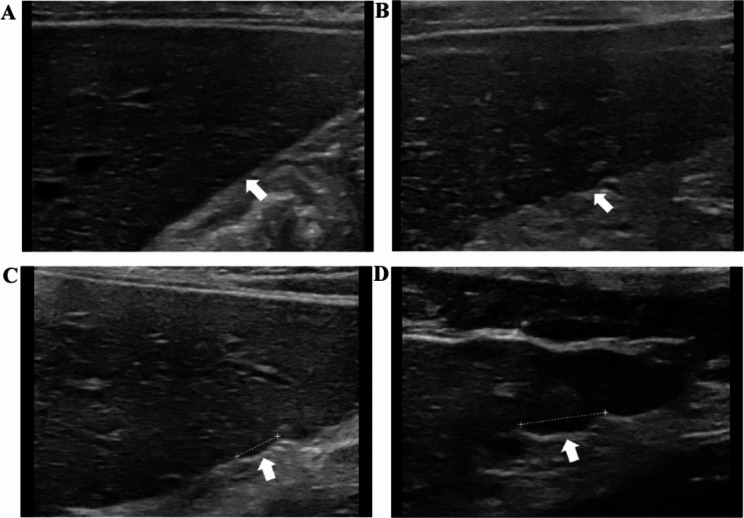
The ultrasound findings of the liver surface. (A) Smooth; (B) mild irregular (≤ 5 mm); (C) moderate irregular (6-10 mm); (D) severe irregular (> 10 mm)
